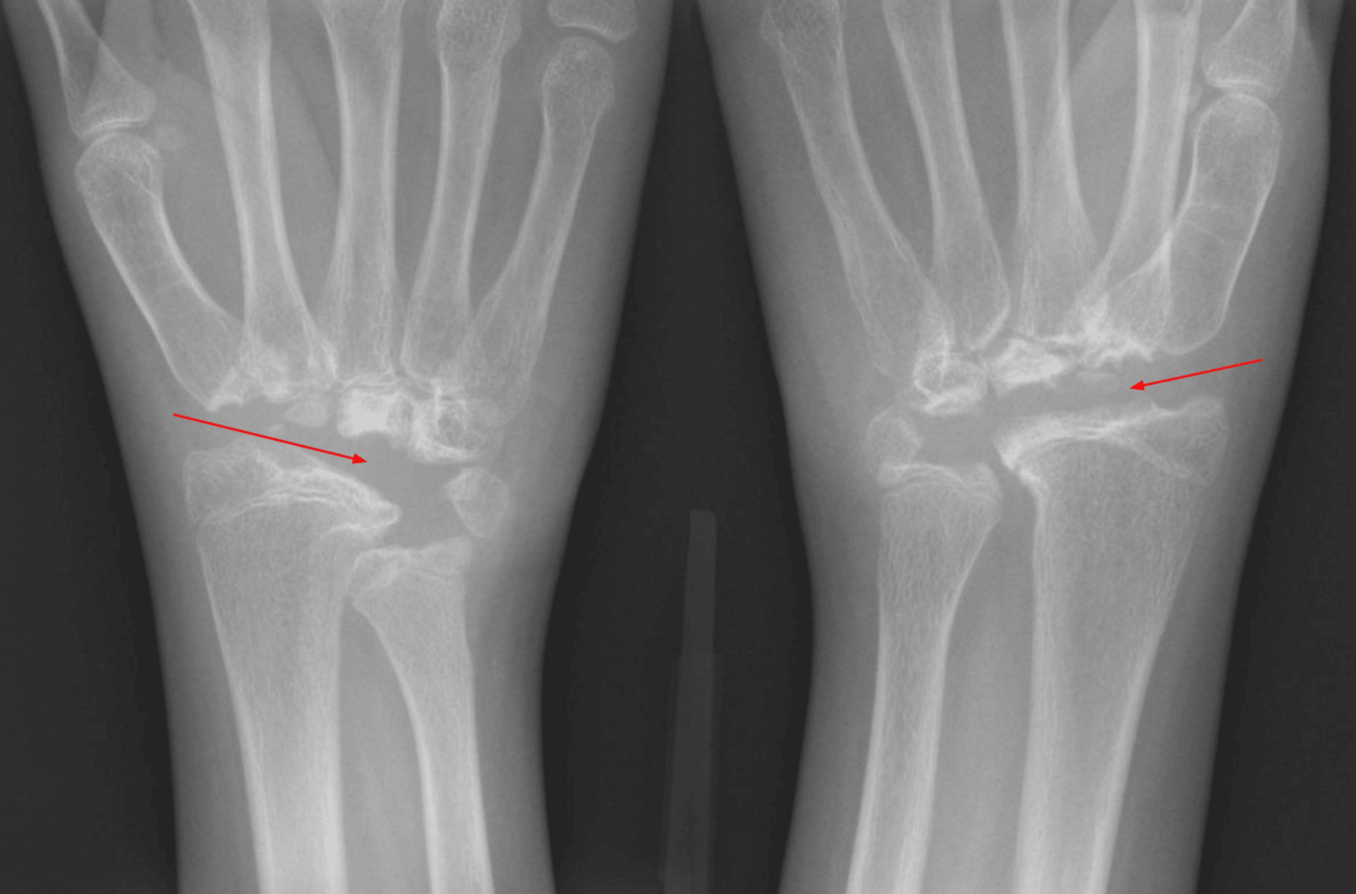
Radiographs of the bilateral wrists show extensive osteolysis of the carpal bones, with degenerative changes at the carpometacarpal joints. Red arrows depict bilateral osteolysis of carpal bones.
